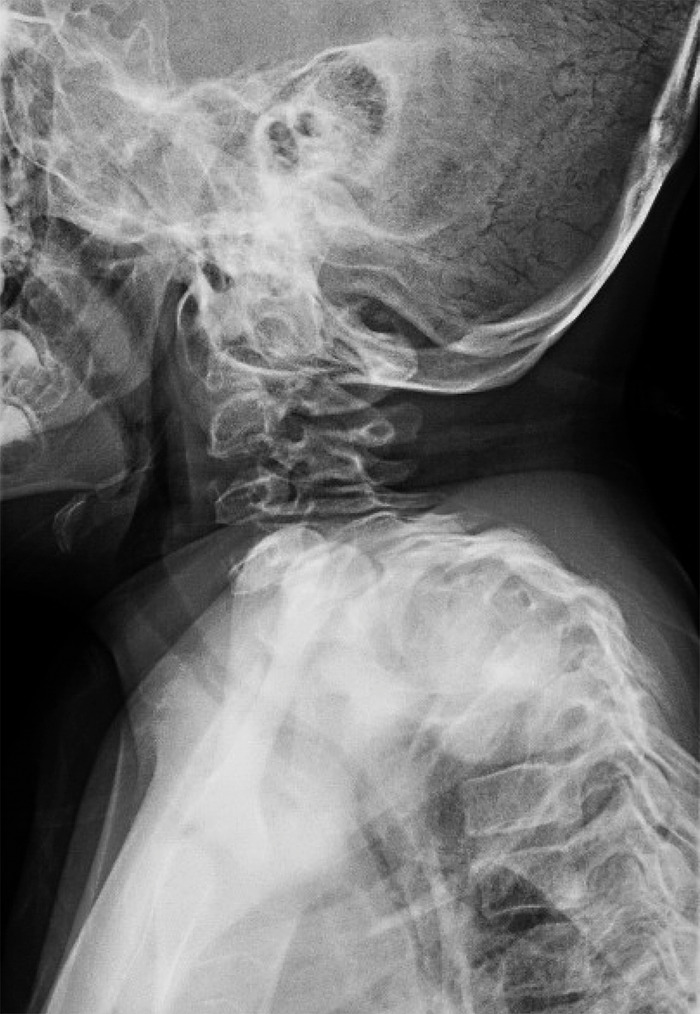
Radiograph of cervical and thoracic spine of the male sibling at age 4 showing severe spinal deformity.
